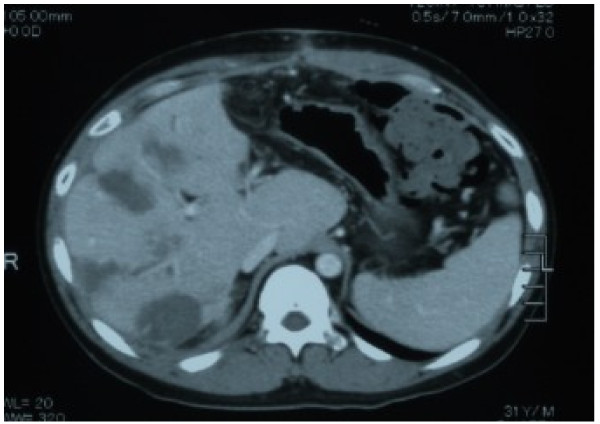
Computed tomography showed multiple low-density areas in the remnant liver following microwave coagulation.
